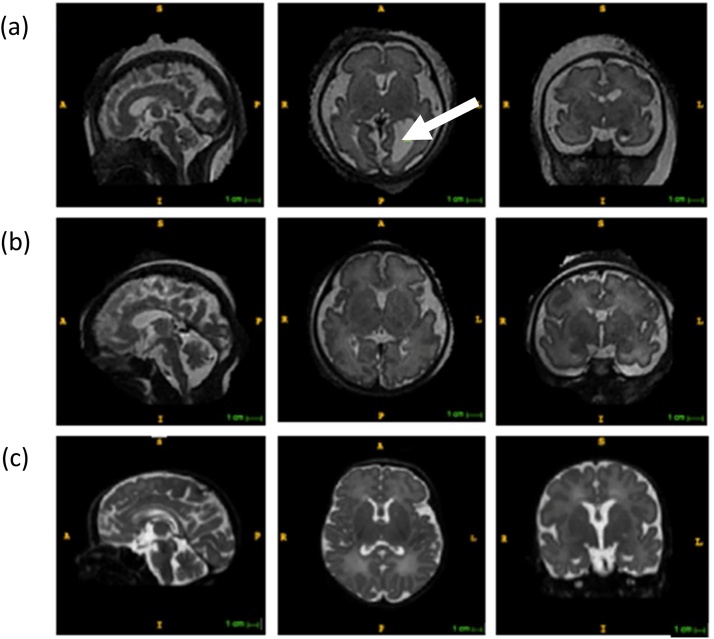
Structural MRI images of fetuses and a neonate with DS after motion correction and reconstruction (SVR) (left to right: sagittal, axial and coronal planes). (a) 30-week-old fetus also demonstrating unilateral ventriculomegaly, enlargement of the ventricles of the brain (shown by arrow); (b) 34 week old fetus; and (c) 43 week old neonate.
